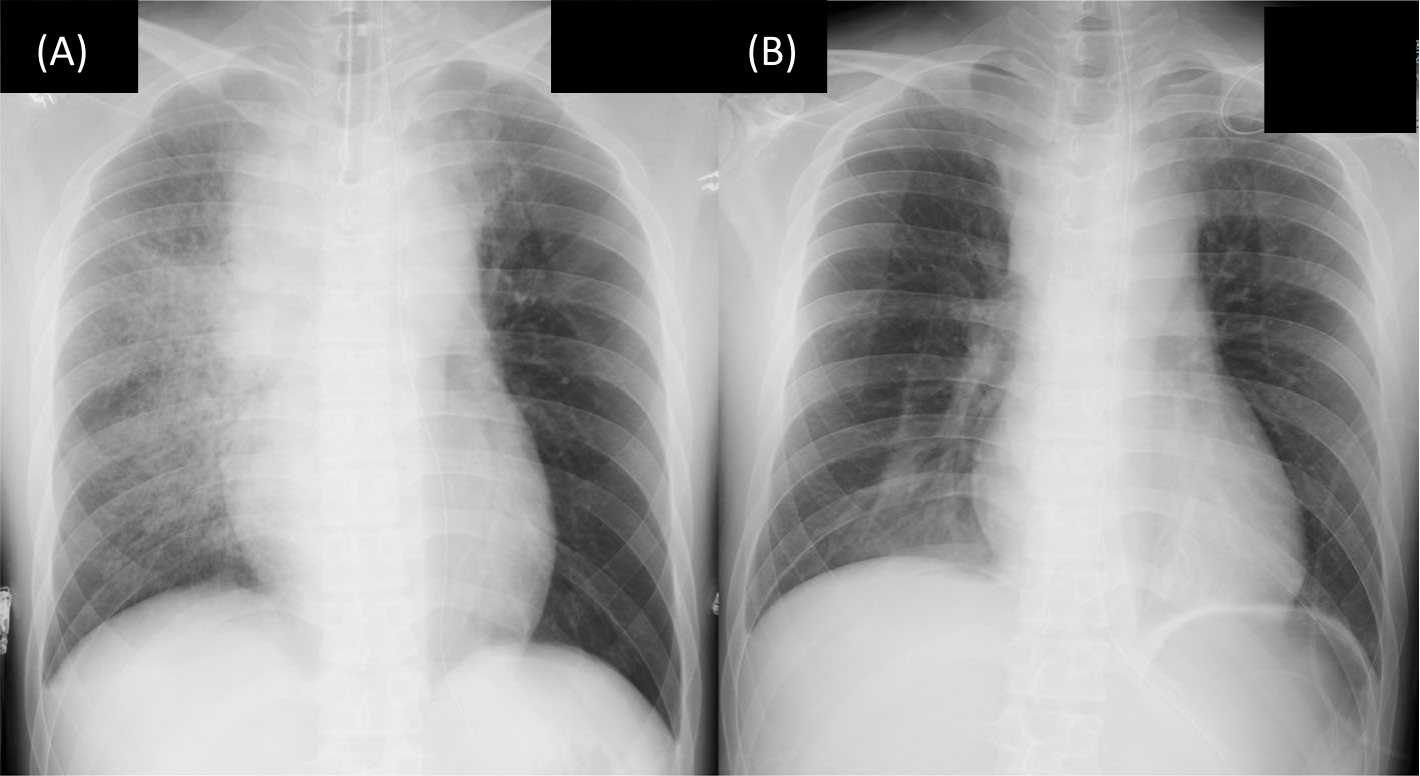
Chest radiography (A) showing diffuse opacities in the right lung due to negative pressure pulmonary edema after postoperative extubation in the supine position, and B repeat radiography on postoperative day 5 showing clearing of the opacities in the right lung after successful extubation in a semi-sitting position
